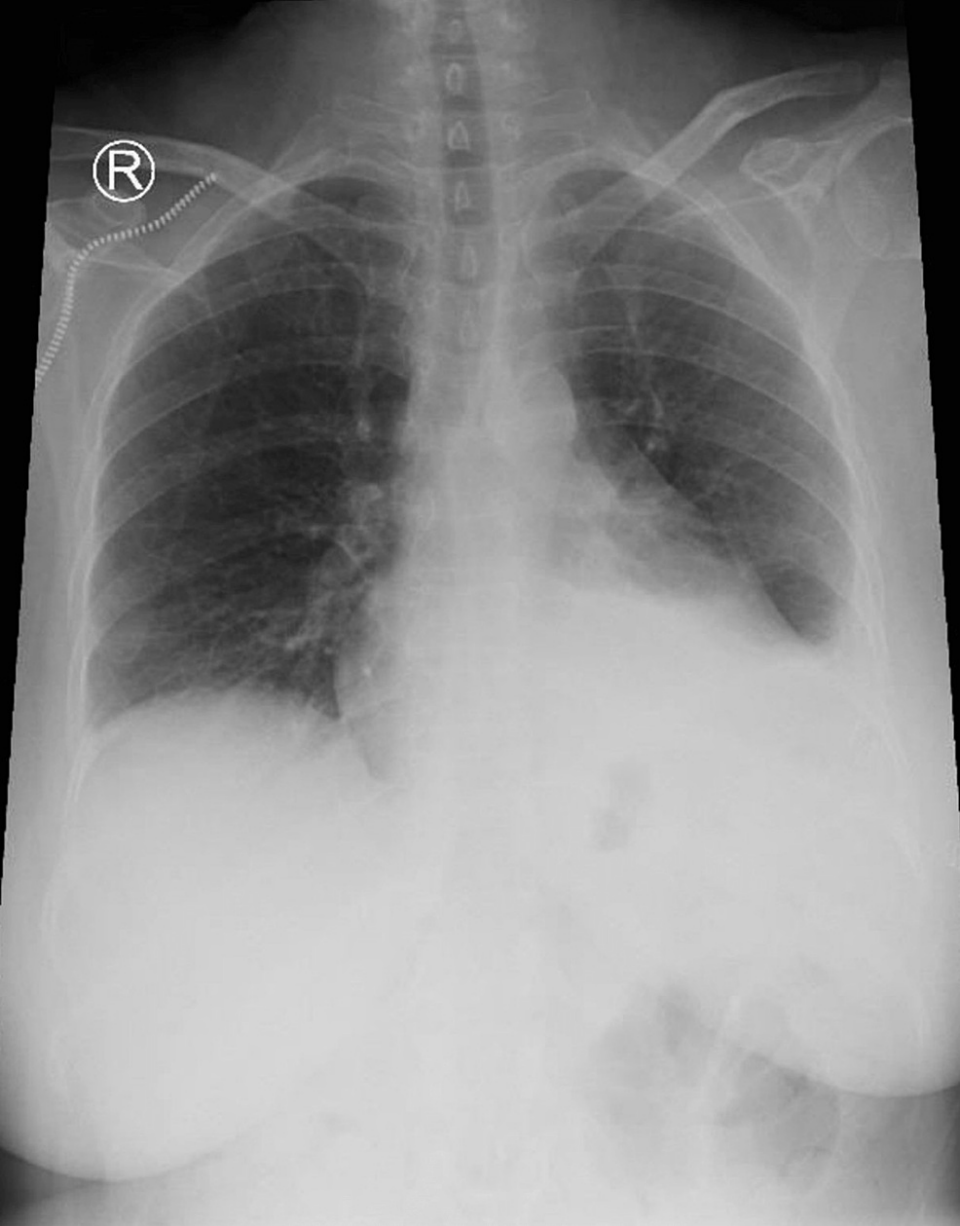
Chest x-ray showed the patient's complete recovery, after seven months of follow-up.
